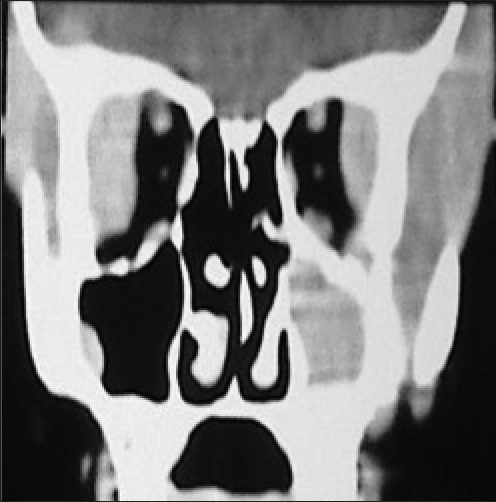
Coronal CT showed the left maxilla also infiltrated by the lesion
